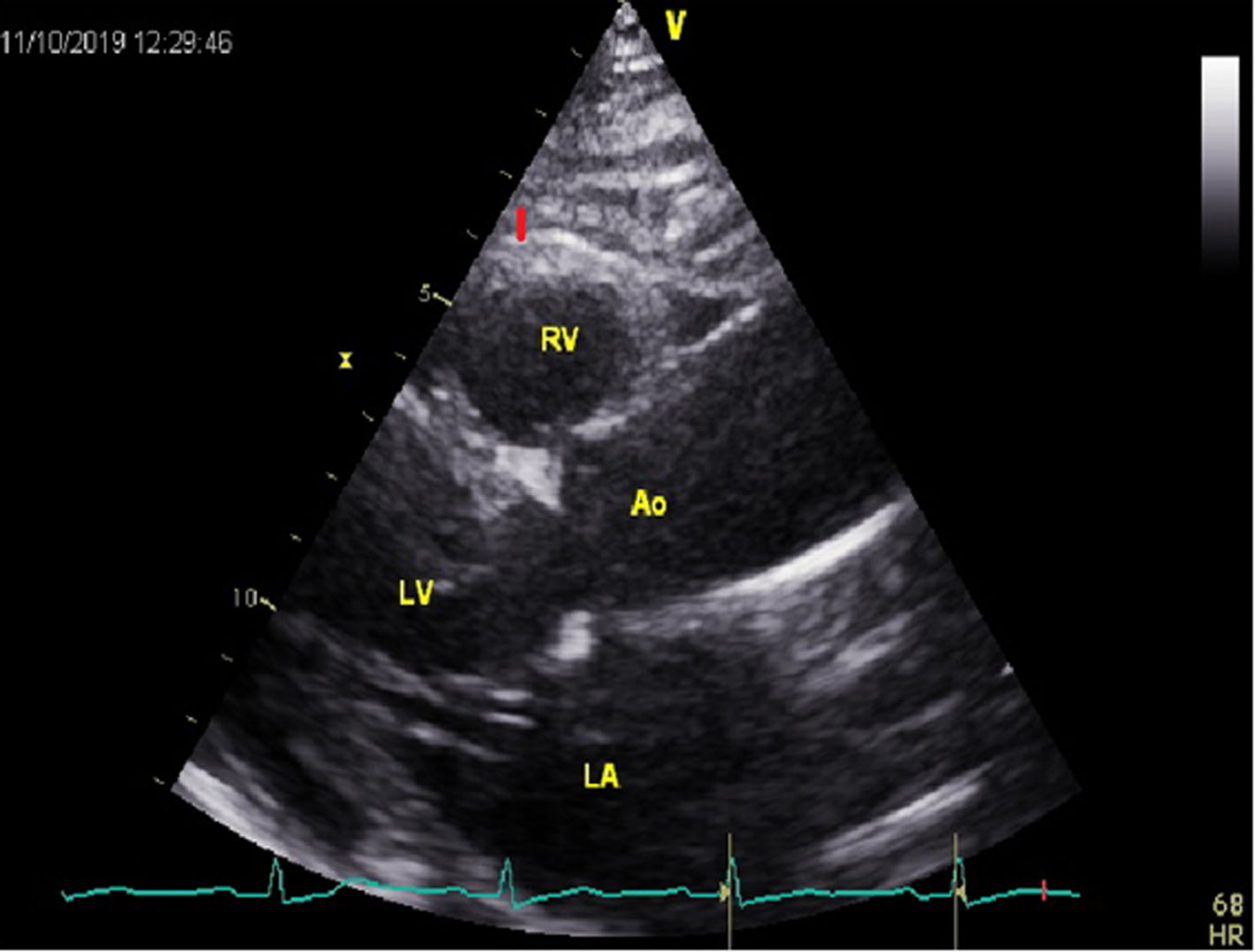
The measurement of thickness. The measurement of thickness of epicardial adipose tissue in parasternal long axis view (red segment). LV-left ventricle, Ao-aorta, LA-left atrium, RV-right ventricle.
